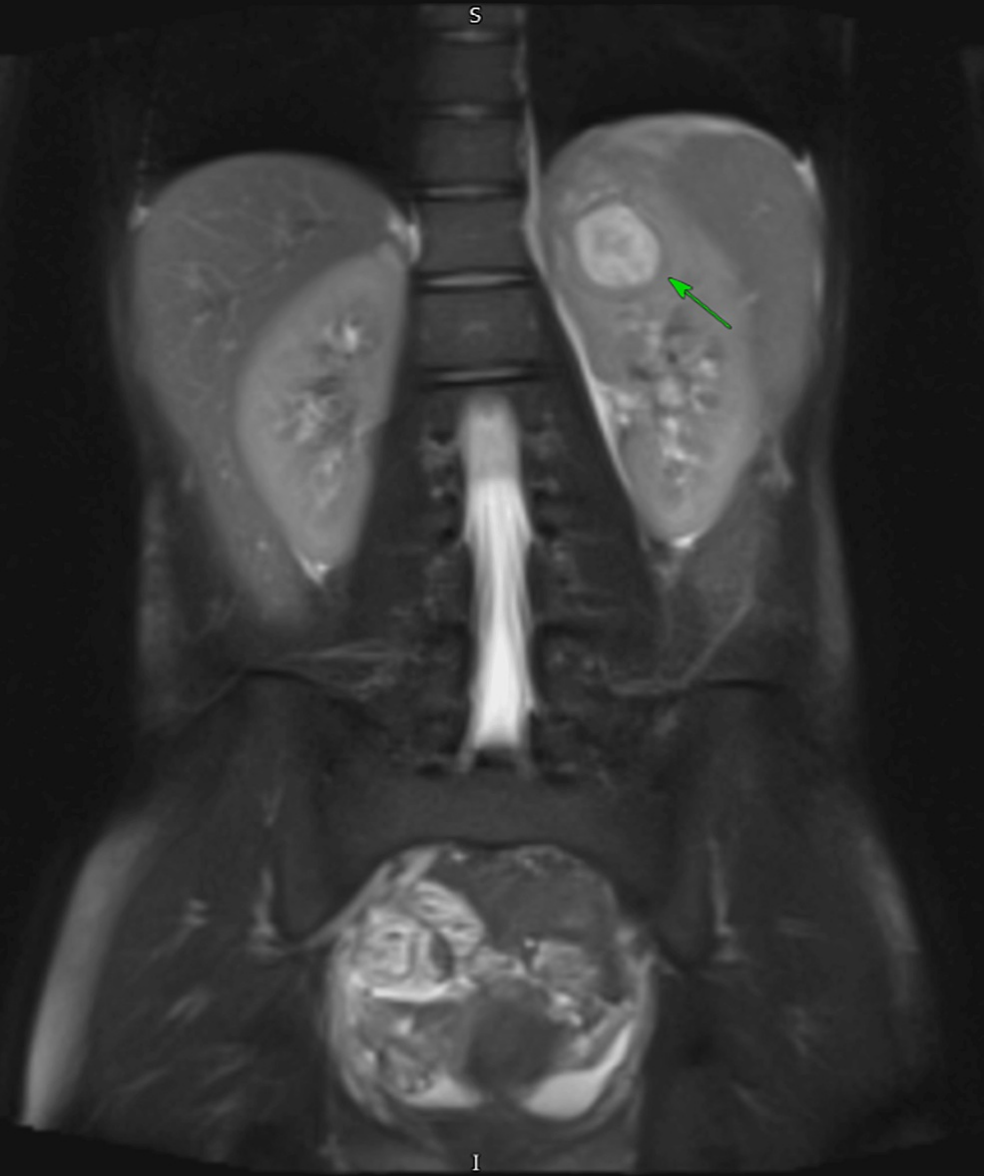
MRI of the left renal abscess
